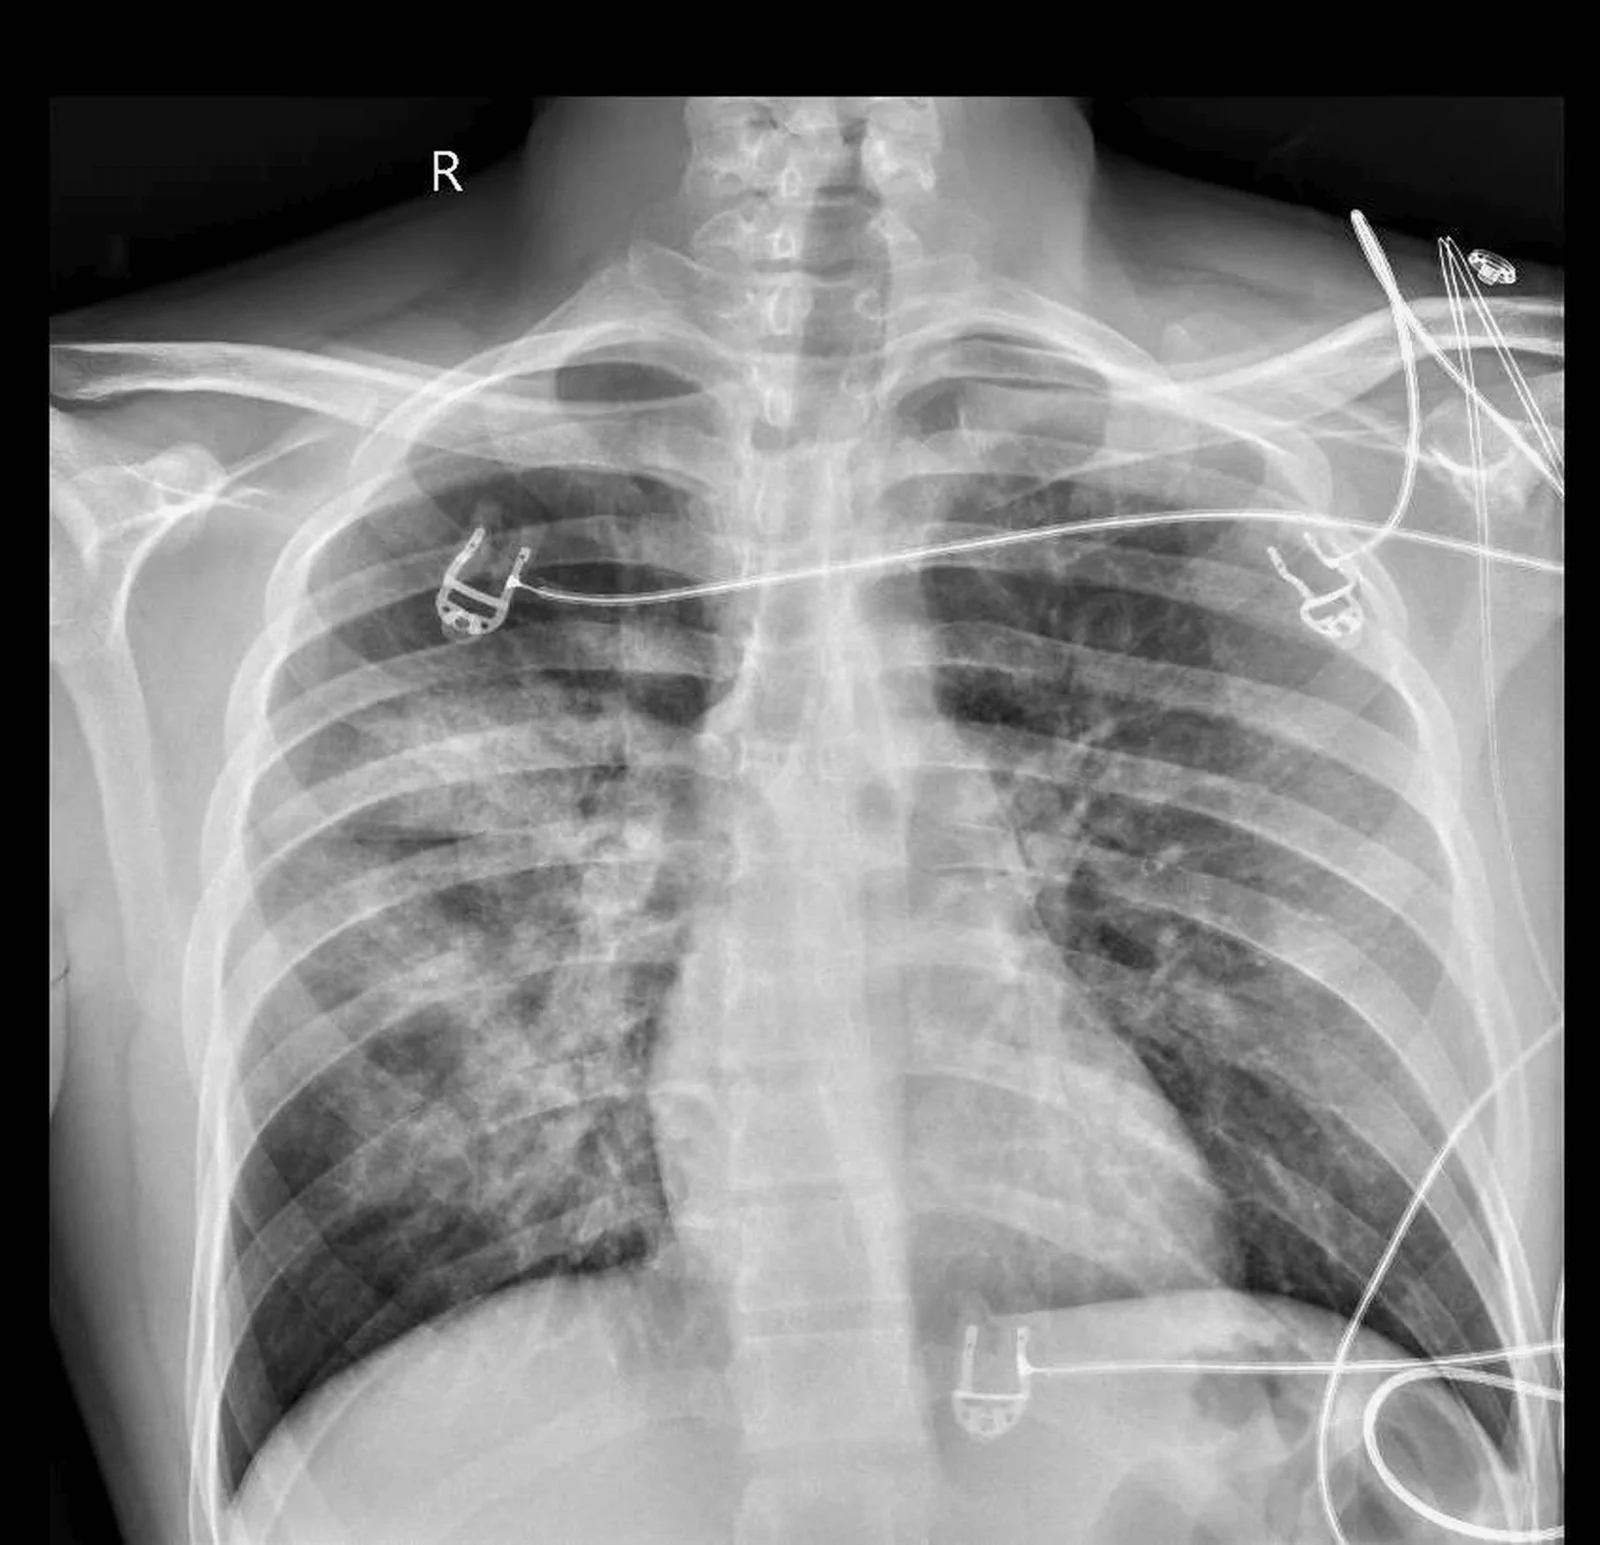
Post-Extubation Pulmonary Air-Space Opacities. Postoperative chest radiograph obtained after laryngospasm and acute respiratory deterioration, demonstrating bilateral patchy central/perihilar air-space opacities, substantially more pronounced in the right lung, without apparent cardiomegaly.
